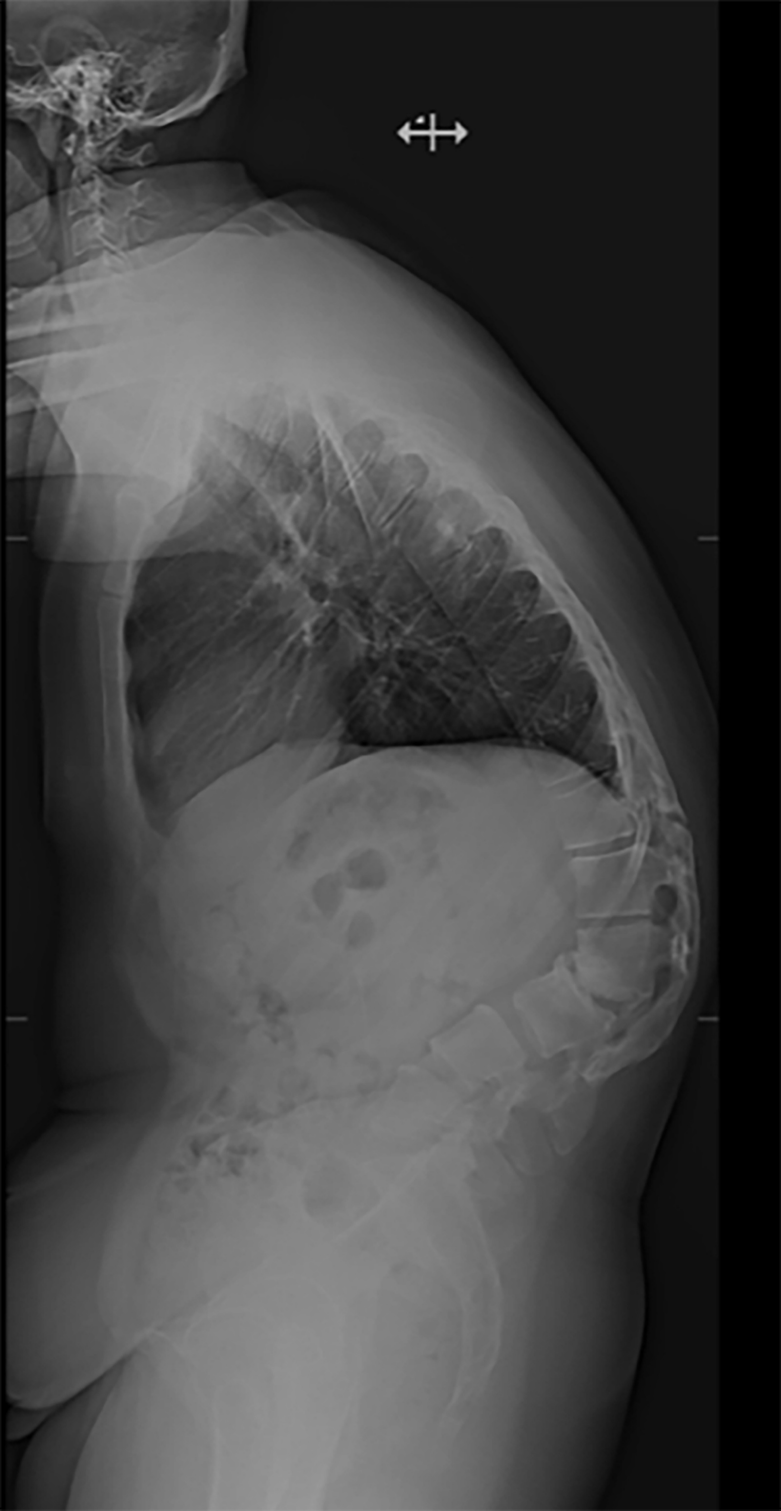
Preoperative Lateral Radiograph Preoperative lateral radiograph demonstrating standing posture and acute kyphosis at L2-L3
